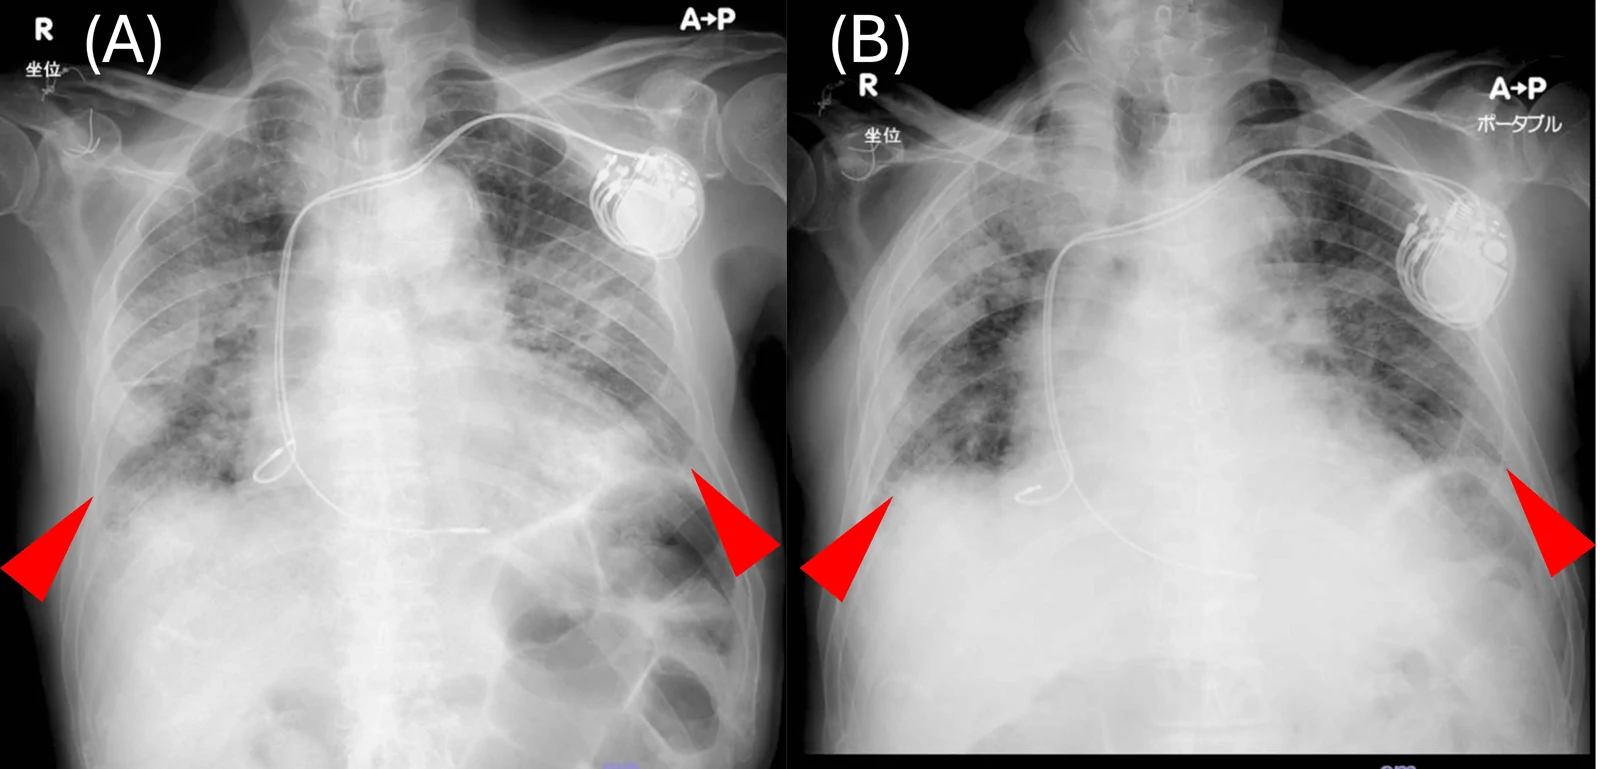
Chest radiographs on admission (A) and hospital day 10 (B) Serial chest radiographs demonstrating increased bilateral pulmonary opacities on hospital day 10 (B) compared with admission (A), highlighted by red arrowheads
